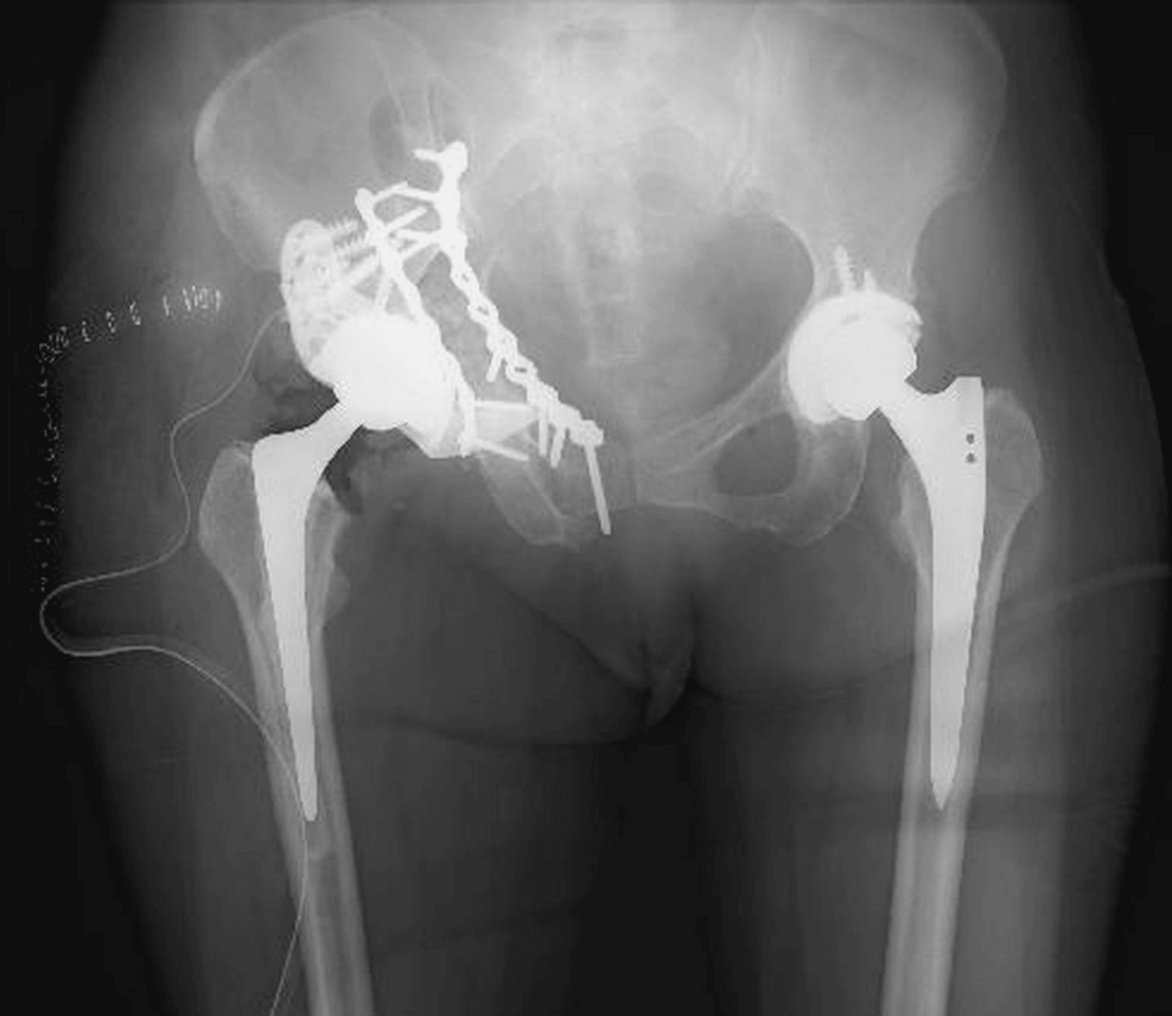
Postoperative plain radiograph of the pelvis (anteroposterior view). Plate fixation was performed from both the anterior and posterior aspects. The acetabulum was reconstructed using a cage, and the femoral stem was revised with a cemented stem.
